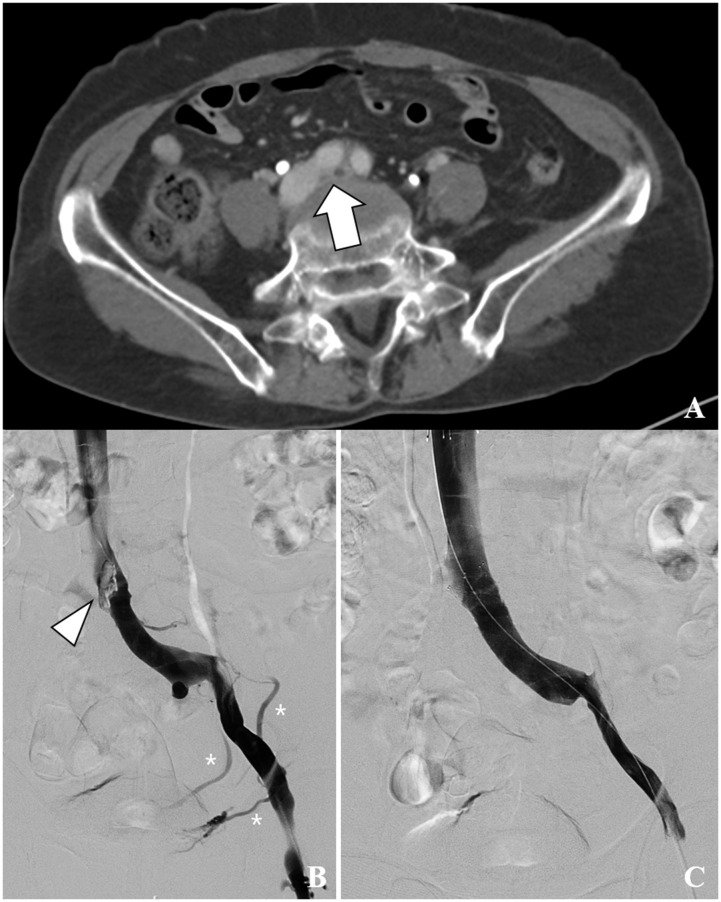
Endovascular treatment of May-Thurner syndrome-associated iliofemoral deep vein thrombosis in a 76-year-old woman. A. Contrast-enhanced computed tomography venography showing compression of the left common iliac vein (LCIV) by the overlying right common iliac artery and L4 vertebral body (arrow), consistent with May-Thurner syndrome. B. Conventional venography reveals a prominent venous spur and > 50% luminal narrowing at the LCIV (arrowhead), along with collateral venous flow (asterisk). C. Post-stenting venogram after the deployment of a 14-mm self-expandable stent demonstrating complete resolution of venous stenosis, spur formation, and collateral flow.
